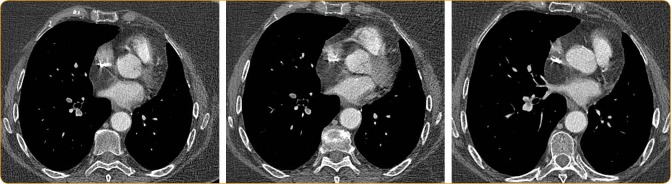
Pulmonary embolism as seen in the vessels in the horizontal slides of the CT scan.
